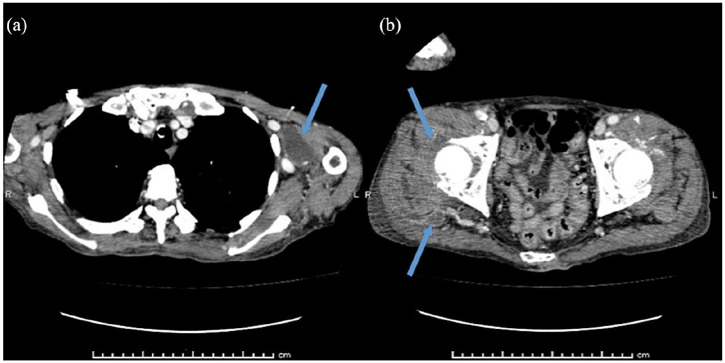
(a) Contrast-enhanced computed tomography (CT) shows a capsule-enclosed abscess in the left axilla (arrow). (b) Contrast-enhanced CT shows two capsule-enclosed abscesses in the right leg (arrows).
